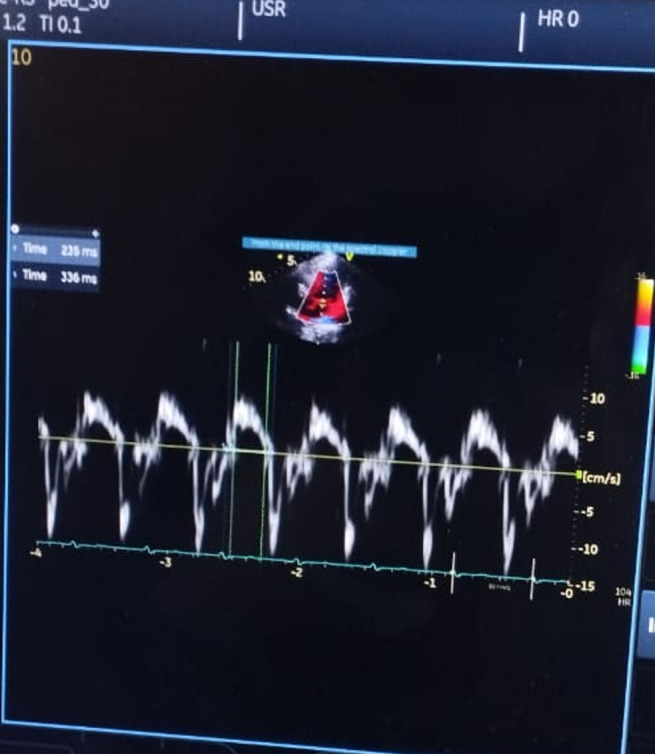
Pulsed Wave Tissue Doppler Imaging After Treatment. Pulsed wave tissue Doppler imaging from the apical 4-chamber view, sampling from the septal mitral annulus in the same child after enzyme Q10 treatment. The E’/A’ ratio has improved to 1.5, and the LV MPI has decreased to 0.42 (336 − 235/235), suggesting an improvement in diastolic and systolic function. This demonstrates the potential beneficial effects of enzyme Q10 therapy in this patient population
